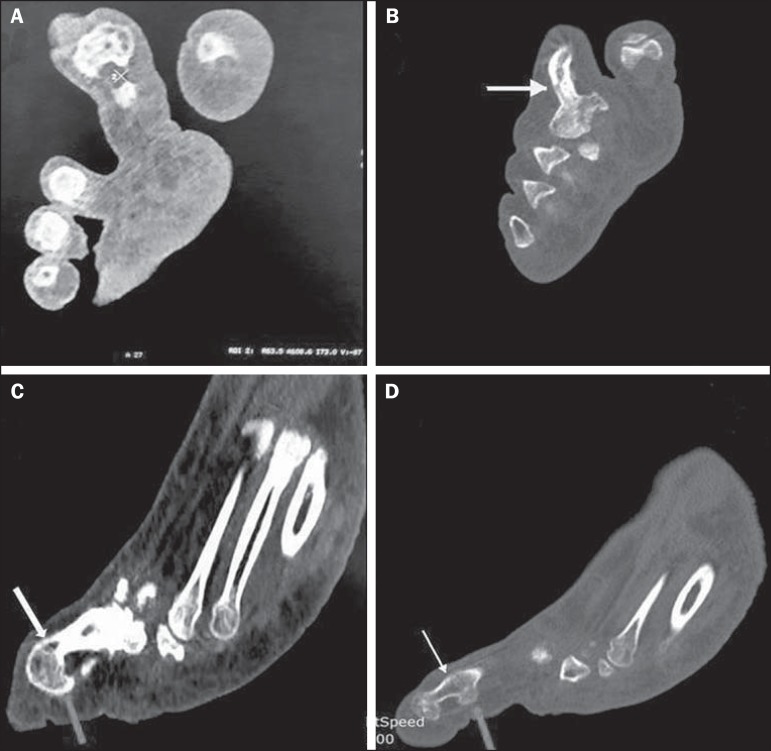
A: Non-contrast-enhanced CT scan, in the axial plane, showing marked
